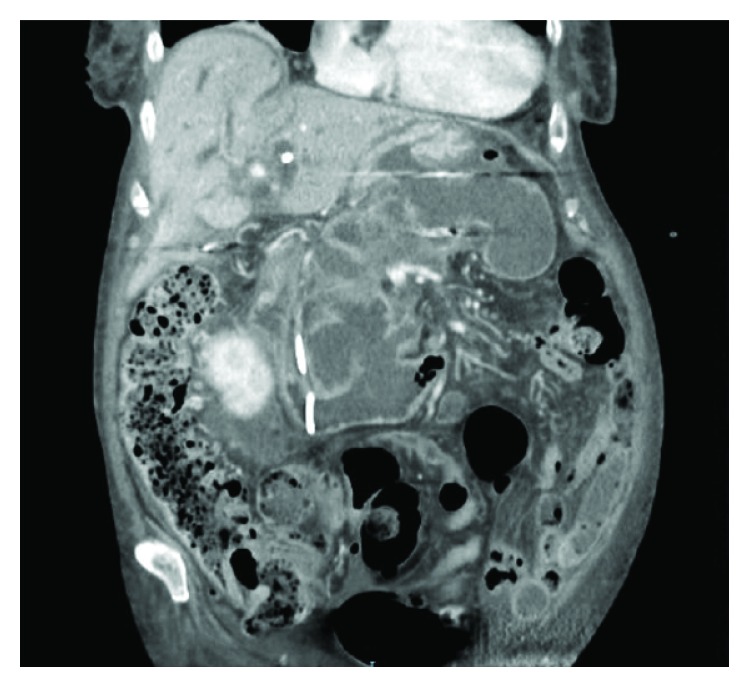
Coronal image of a contrast-enhanced abdominal CT in venous phase showing the rim-enhancing fluid density in the pancreatic bed compressing the stomach against the inferior surface of the liver; and communicating to the stomach via a 1.5 cm defect in the greater curvature.
